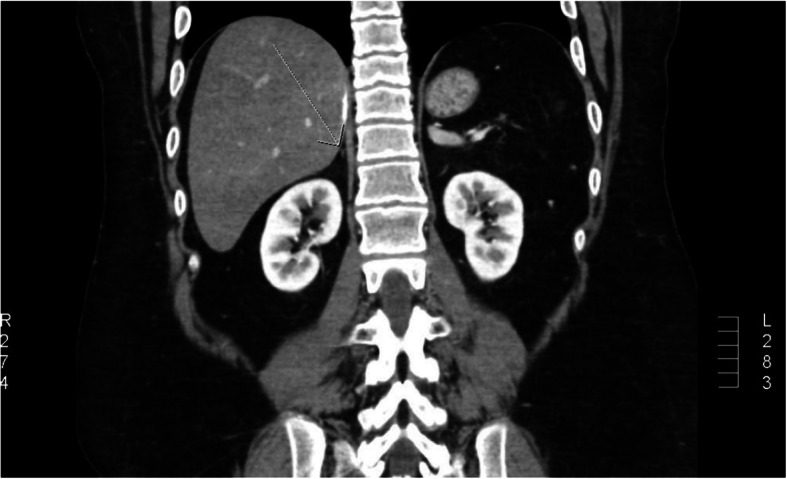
Abdominal CT scan results showing severely atrophied adrenal glands (arrow)
